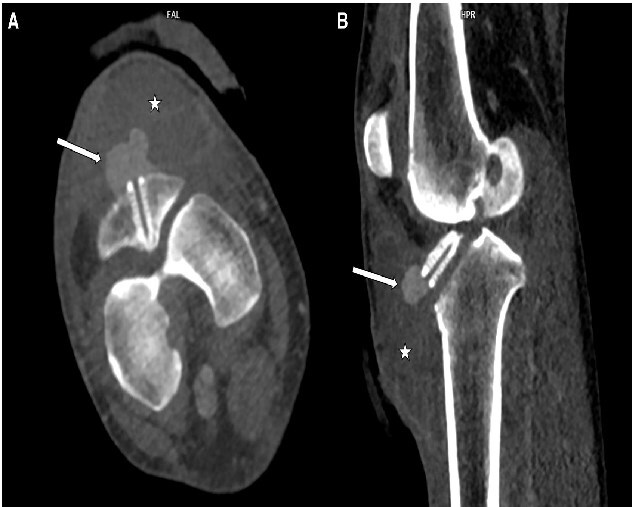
Axial (A) and sagittal (B) reconstruction of the CT angiography showing a haematoma (white star) and a hyperdense pseudonodular lesion in direct contact with the distal part of the tibial tunnel screw, compatible with a pseudoaneurysm.
